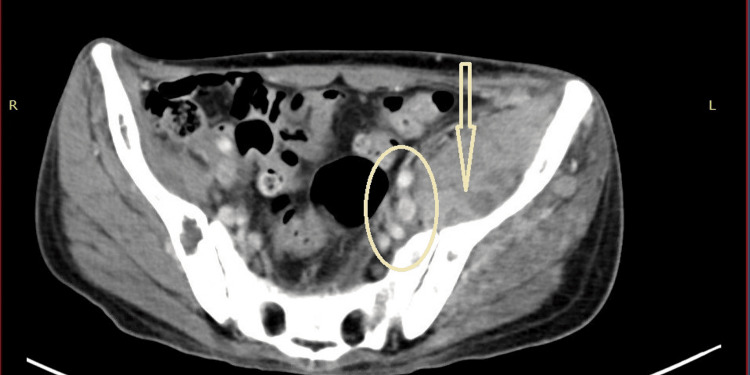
CT scan abdomen The arrow indicates a large soft tissue mass involving the left iliac fossa; the circle indicates left pelvic lymphadenopathy.
